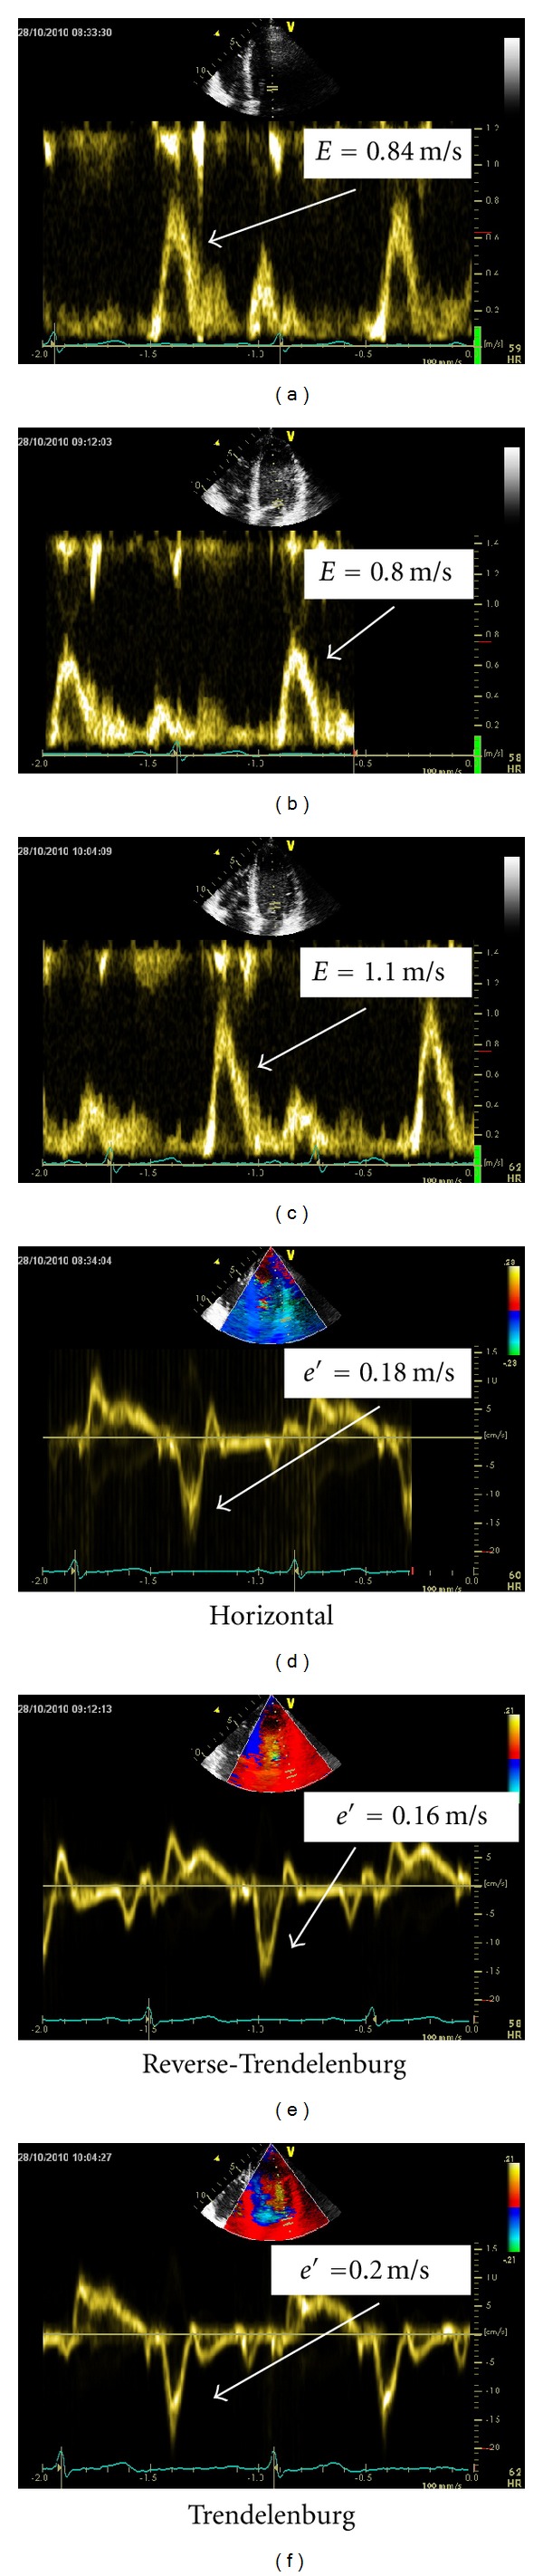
Examples of echocardiographic indices of diastolic function in the horizontal position, reverse-Trendelenburg position, and the Trendelenburg position during positive pressure ventilation. *e*′: peak early diastolic velocity of the lateral mitral annulus, obtained with tissue Doppler. *E*: peak early transmitral blood flow.
